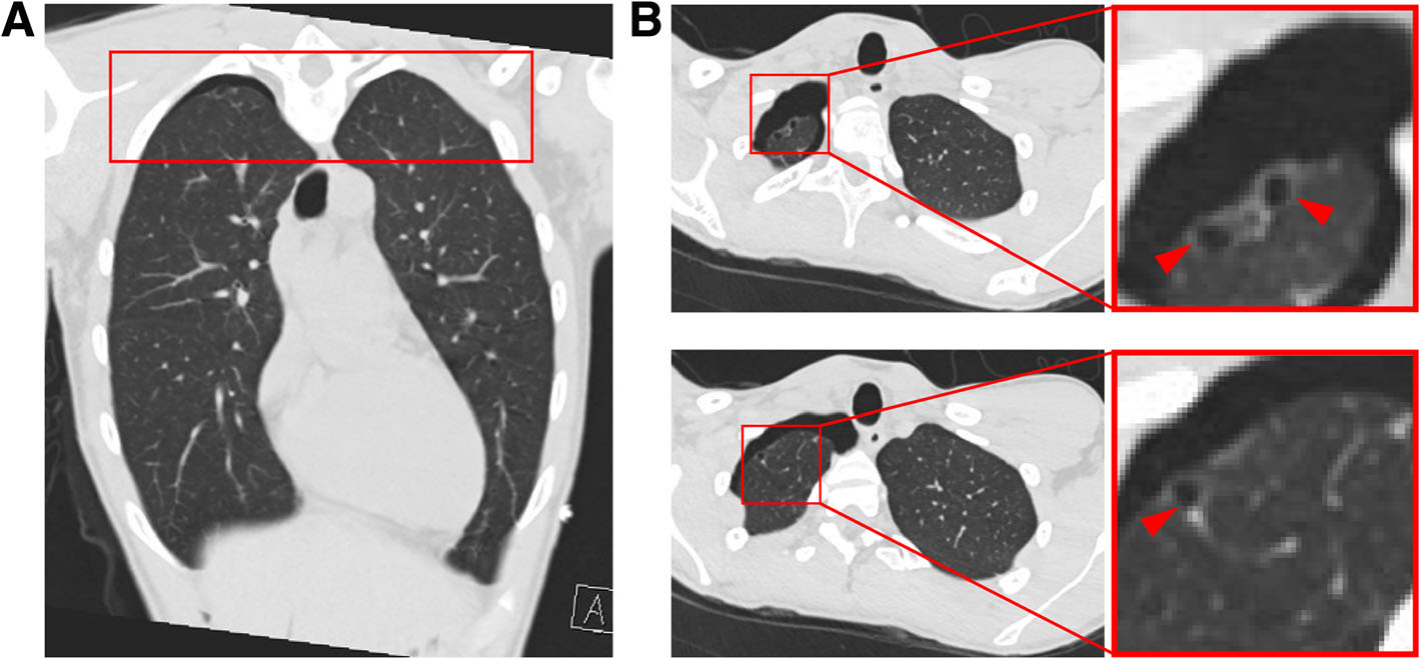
Coronal (a) and axial (b) computed tomography slices showing apical blebs and residual pneumothorax. Axial cuts selected from the *red box* in *panel A*. *Insets* from axial images highlight the presence of apical blebs (*arrowheads*)
